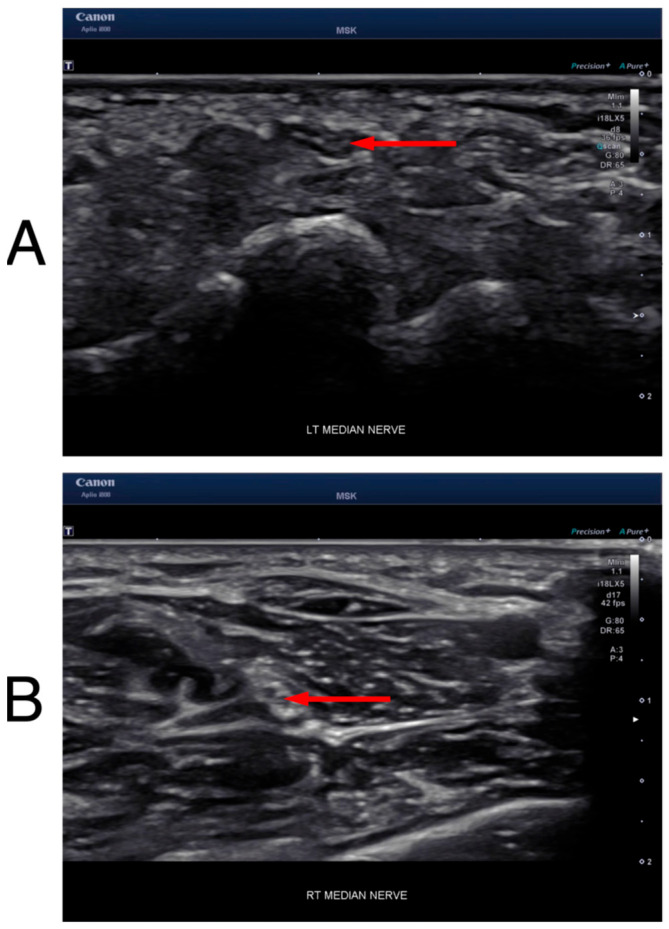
Sample frame from ultrasound video taken from (A) wrist crease and (B) distal forearm. The location of the median nerve is denoted by the red arrow.
